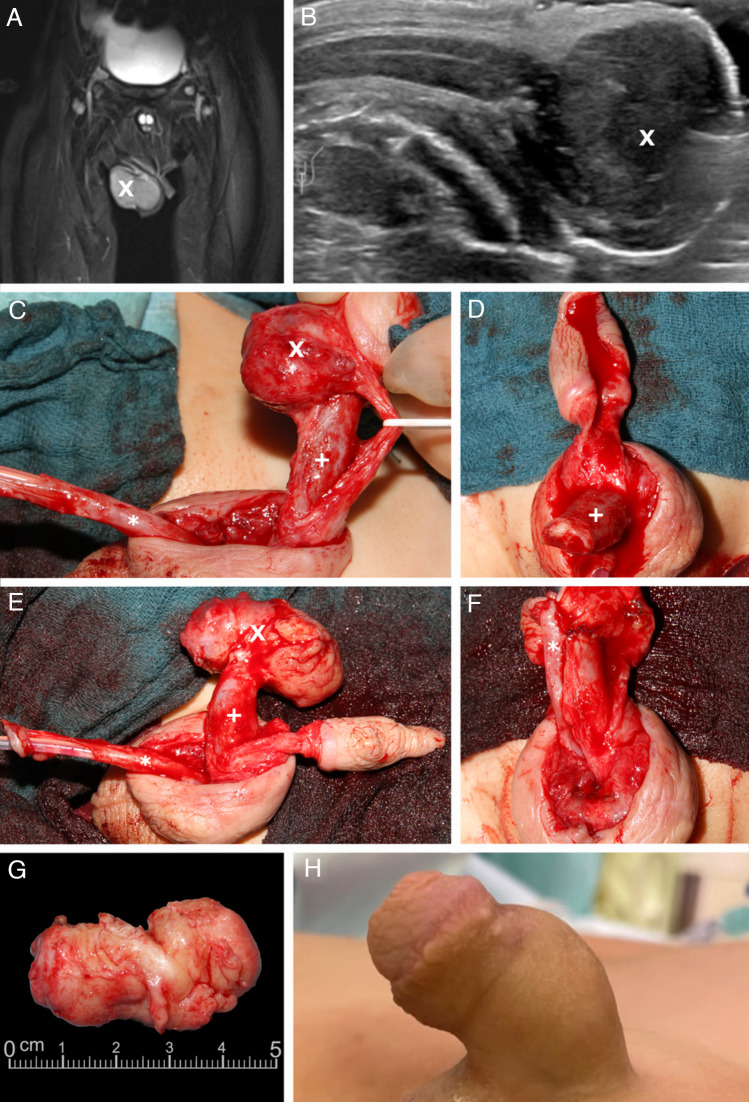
A 26-month-old boy with neuroblastoma stadium 4 and a metastasis to the penile shaft. MRI post-contrast with fat saturation, coronary (A). Sonographic longitudinal view demonstrating the relation of the metastasis to the corpus cavernosum (B). Surgical site (C, D, E, F). The resected metastasis (G). Postoperative outcome 18 months after metastatic surgery (H). X = Penile metastasis. +  = Corpora cavernosa. * = Urethra.
